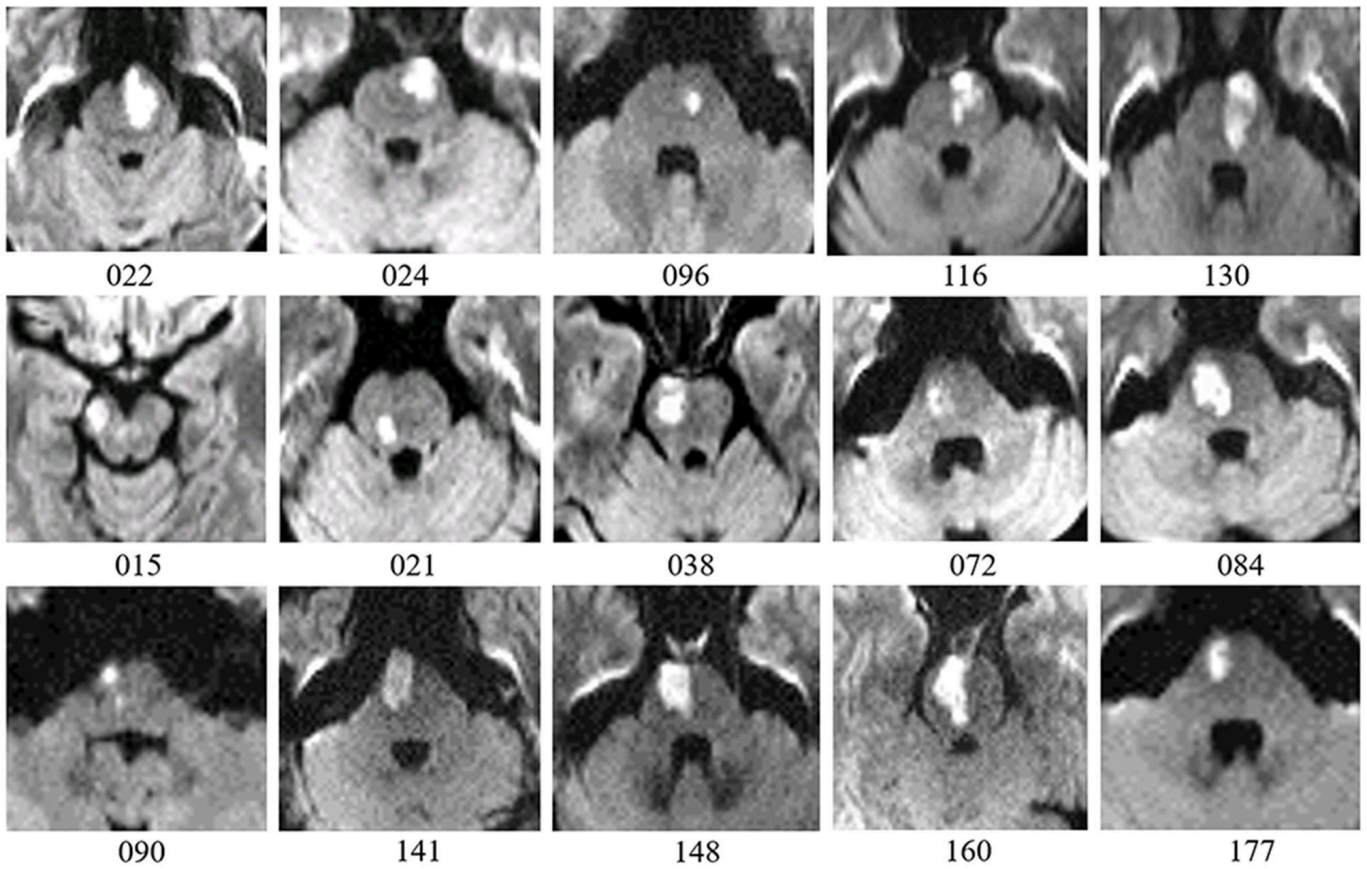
Lesion details of patients with pontine infarction are depicted in axial DWI maps. Number below each image is the participant ID presented in Table 1. Five patients with lesions on the left side are placed on the first row, while 10 patients with lesions on the right side are shown on the following 2 rows. For all images, right is displayed on the left (radiological convention).
